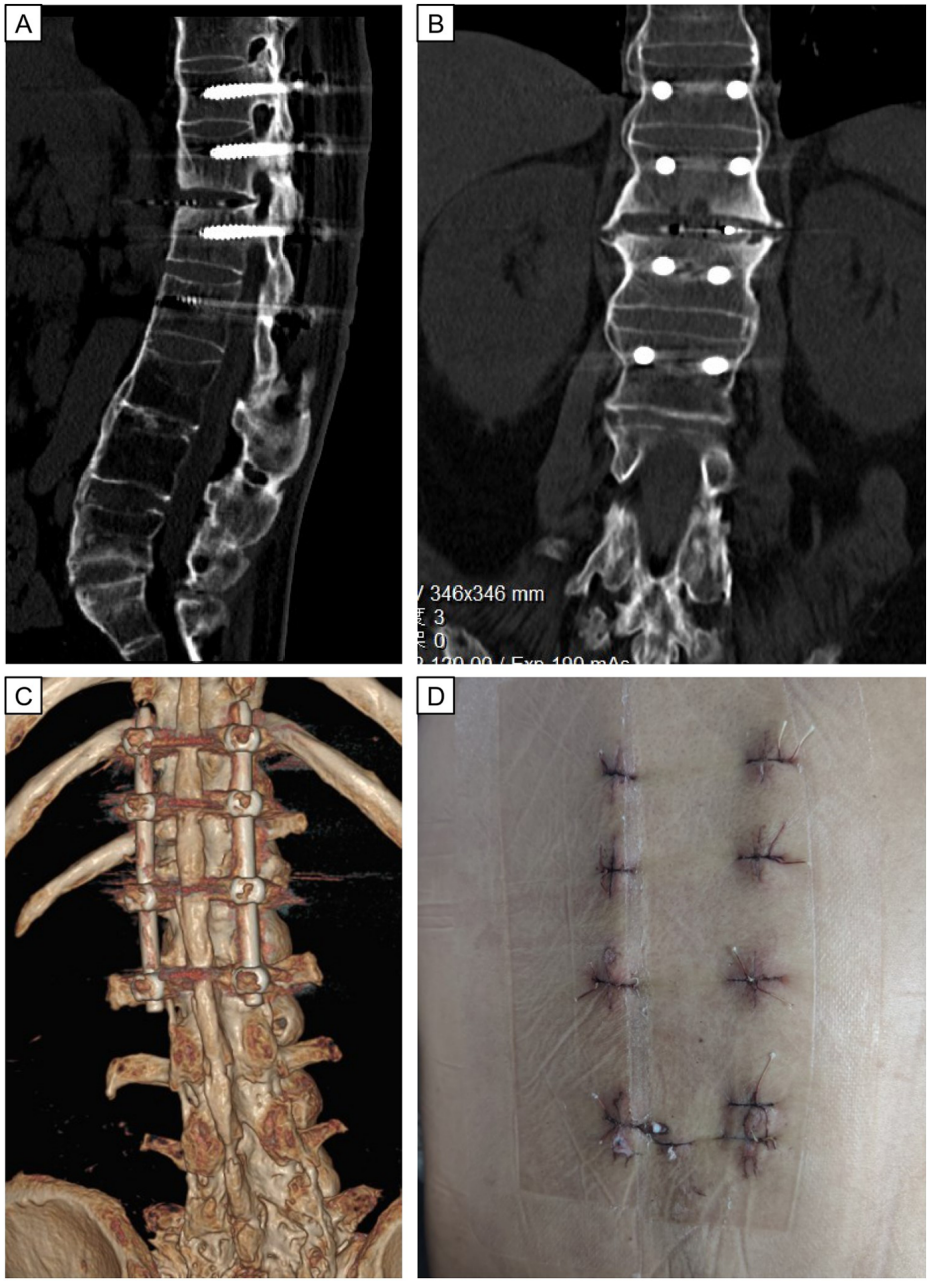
Postoperative immediate CT images and wound scars after minimally invasive surgery of LIF. Postoperative CT images (A–C) show good positioning of the internal fixation and normal sagittal sequence of the lumbar spine. Eight minimally invasive incisions (D) for internal fixation and fusion surgery.
